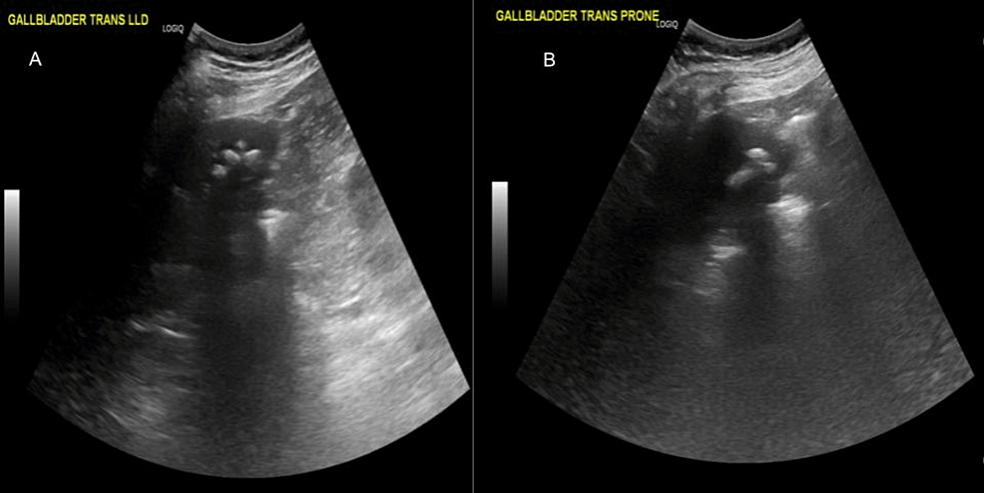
The ultrasound of the abdomen shows multiple stones within the gallbladder without evidence of acute cholecystitis.
